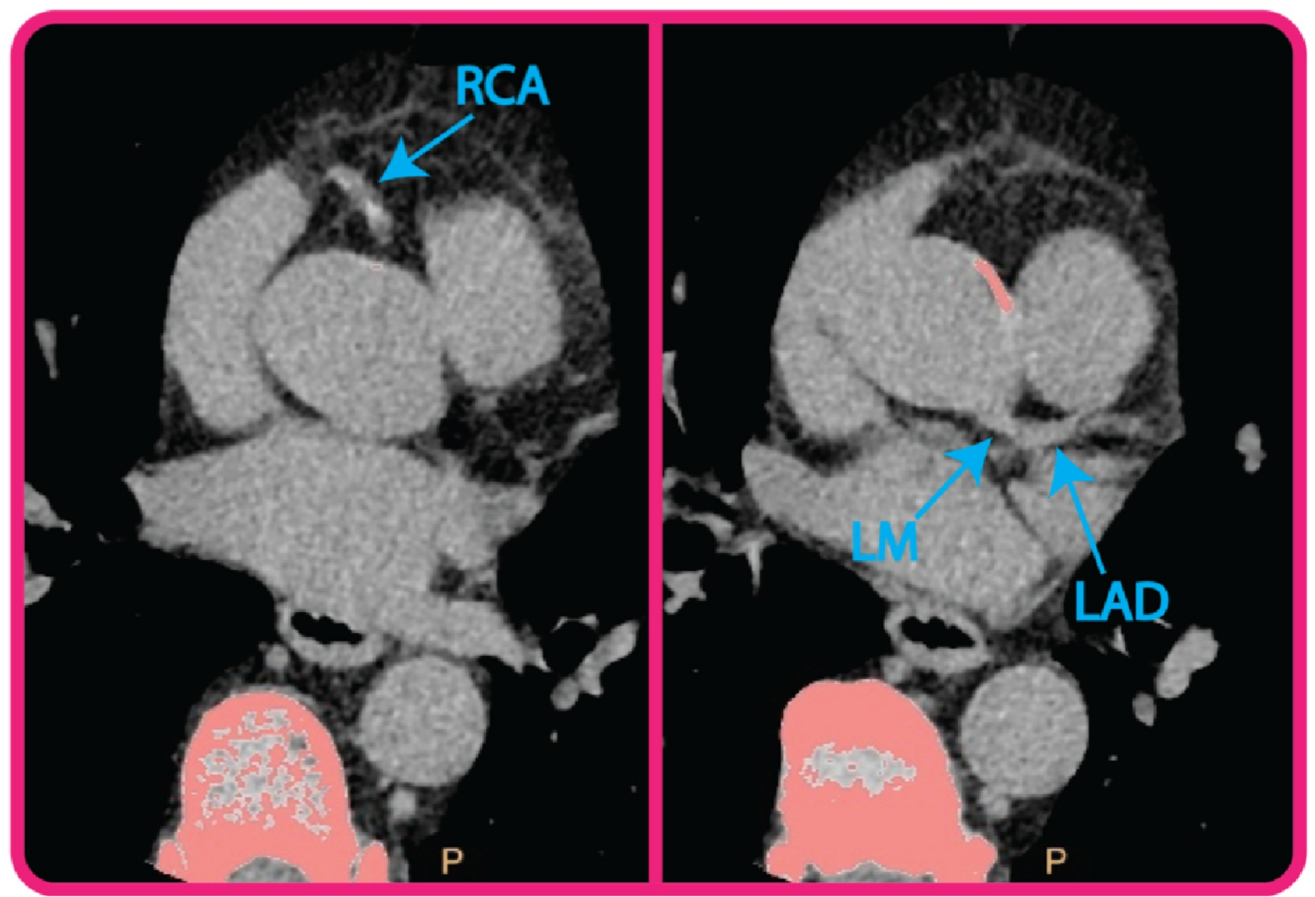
Cardiac computed tomography (CT) results. Pictured are two CT transverse slices from 2018 (patient age: 72) demonstrating the absence of calcification in the right coronary artery (RCA) and left main (LM) and left anterior descending (LAD) coronary arteries.
